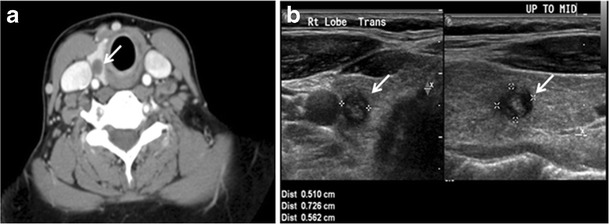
A 51-year-old female patient post left hemi-thyroidectomy, with incidentally discovered right thyroid colloid nodule on CT scan. a Enhanced axial CT scan of the neck demonstrates a well-defined, hypodense right thyroid nodule (*white arrow*) with no internal calcifications or cervical lymphadenopathy. b Transverse greyscale thyroid ultrasound demonstrates a well-defined, hypoechoic right thyroid lobe nodule with a central echogenicity including comet tail (ring down) artefacts (*white arrow*). No vascularity (not shown) or calcifications were detected
